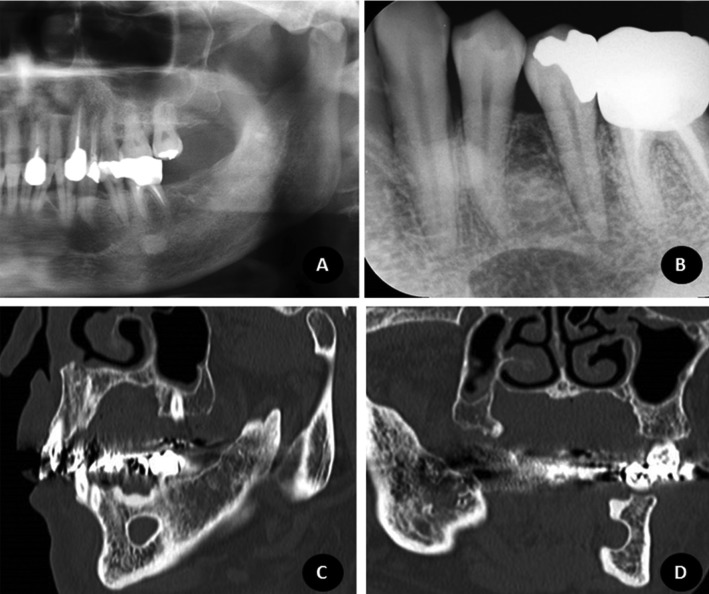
An example of an SBD case in the premolar area from the sample. Figure 3A shows the panoramic radiograph detail of the case; Figure 3B the periapical radiograph; Figure 3D and 3E demonstrate sagittal and coronal slices in multislice CT.
